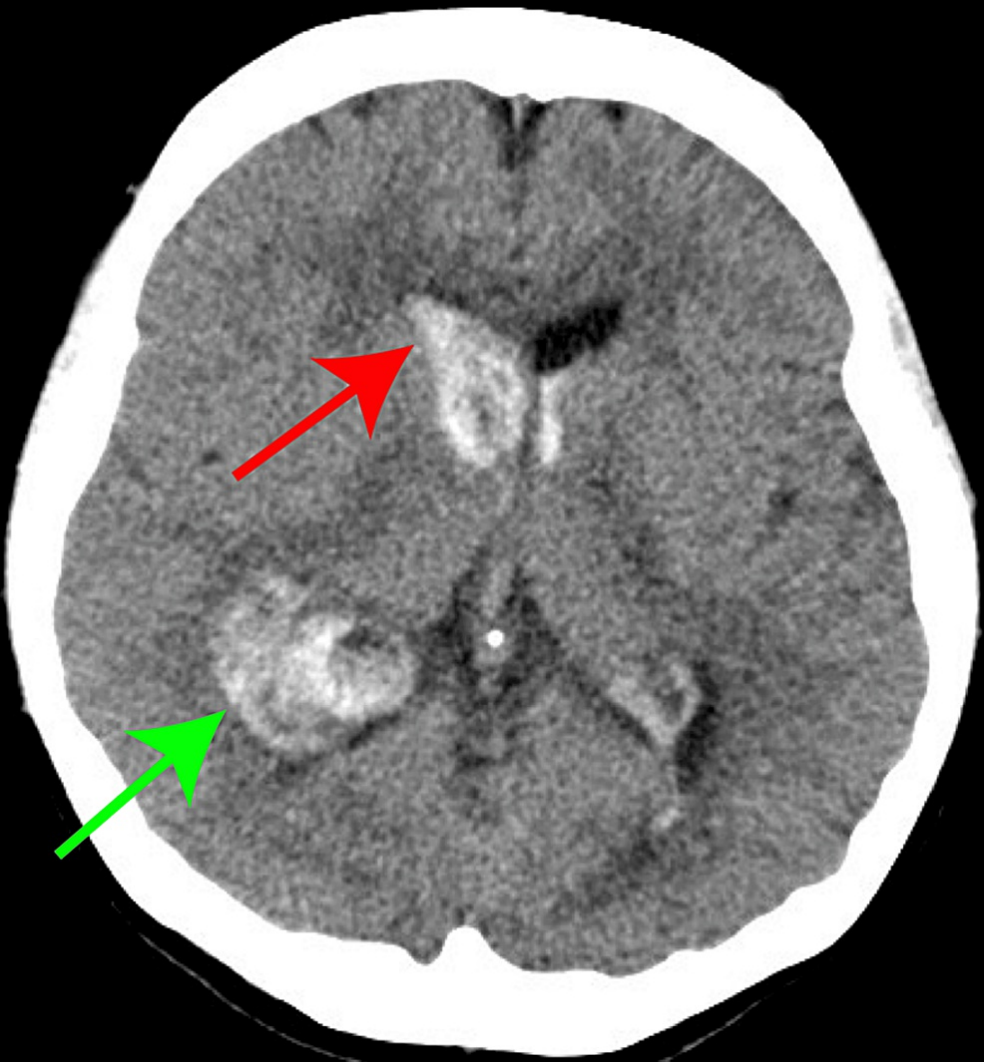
Computed tomography images Intracerebral hemorrhage in the occipital lobe was observed (green arrow), and intraventricular perforation was observed (red arrow).
